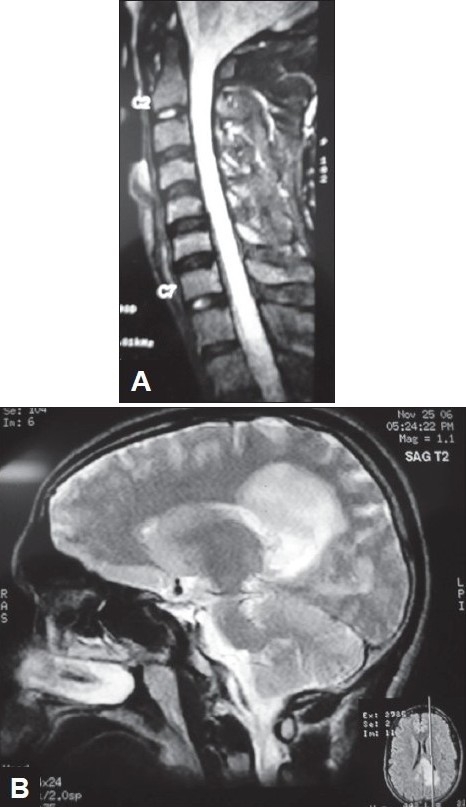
(A and B) 31 year old male presented with three episodes of recurrent myelitis interspersed with one episode of optic neuritis over a period of 18 months. Initial MRI brain was normal. MRI of the cervical cord showed (Fig. 3A) on T2 W image, a linear contiguous lesion spanning more than 3 vertebral segments suggestive of longitudinally extensive transverse myelitis. On his fourth hospital admission, he presented with headache, disorientation and worsening of lower limb power. MRI brain (Fig. 3B) showed large lumpy posteriorly situated subcortical lesions.
